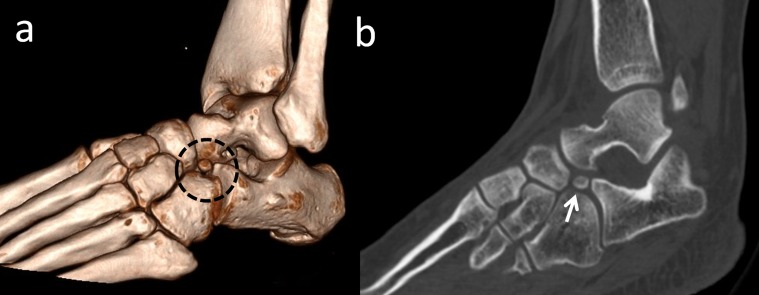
Os calcanei secundarium (a) 3D computed tomography (CT) appearance in the black dotted circle; (b) 2D CT appearance, the white arrow is os calcanei secundarium.
